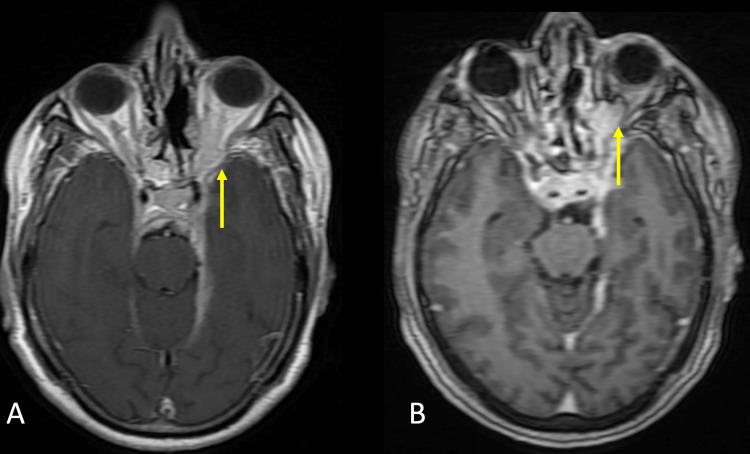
Magnetic resonance imaging with contrast A. Axial T1WI fast spin echo contrast sections with a yellow arrow pointing at left orbital involvement. B. Axial T1WI contrast section with a yellow arrow pointing at left orbital involvement.
